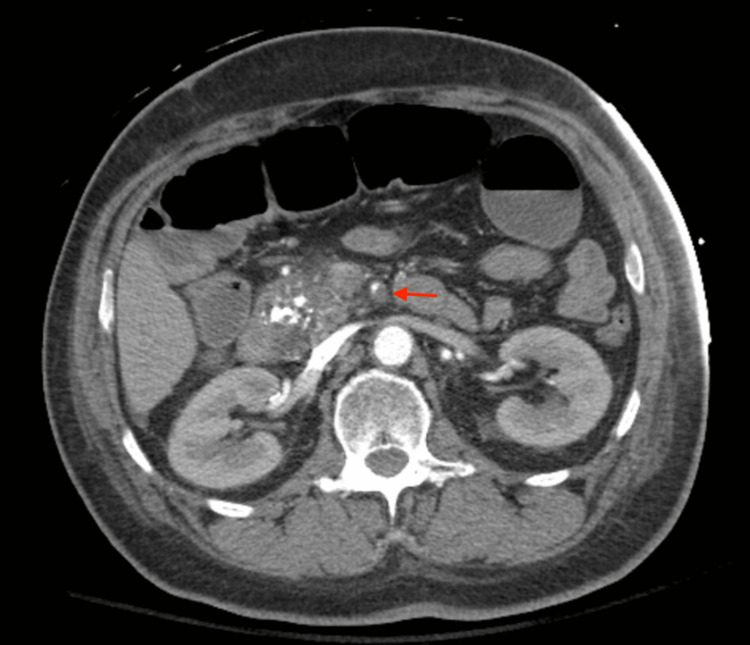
CTA showing a filling defect in the SMA (red arrow), suggestive of SMA occlusion CTA: Computed tomography angiography; SMA: Superior mesenteric artery.
